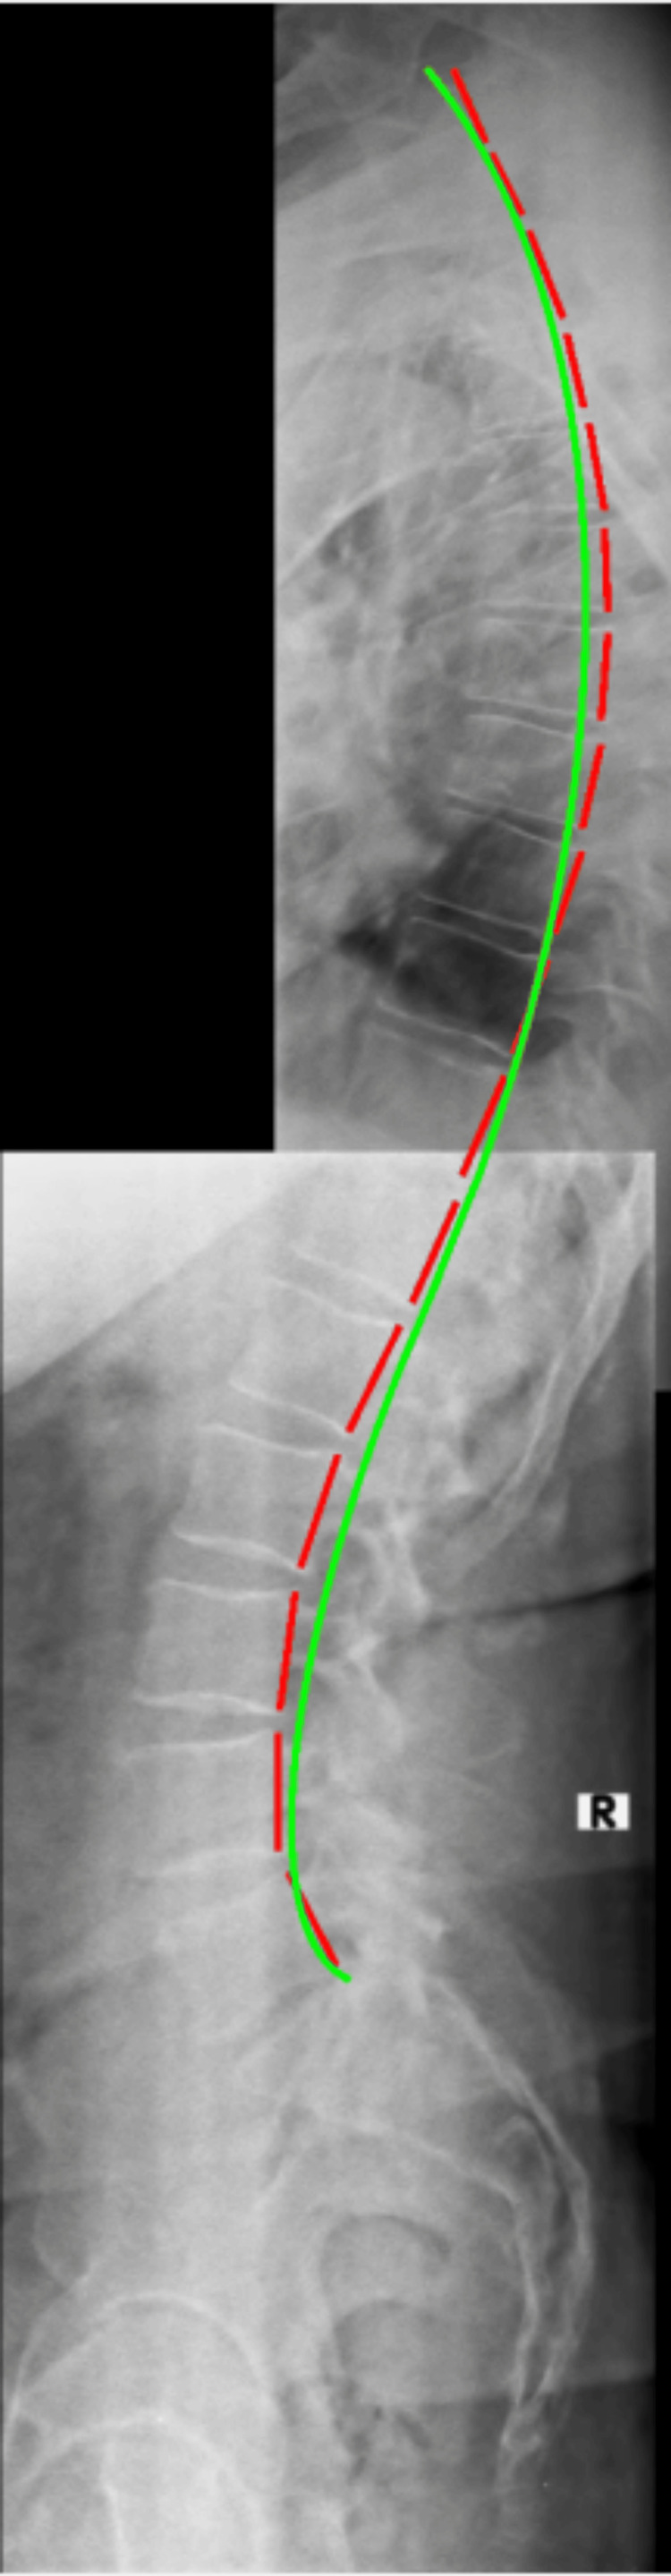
Re-examination lateral thoracic and lateral lumbar radiographs stitched Lateral thoracic and lumbar radiographs demonstrated significant improvement in spine alignment and sagittal balance. The dashed red line measures vertebral body posterior tangents and the green line represents ideal thoracic and lumbar curvature.
